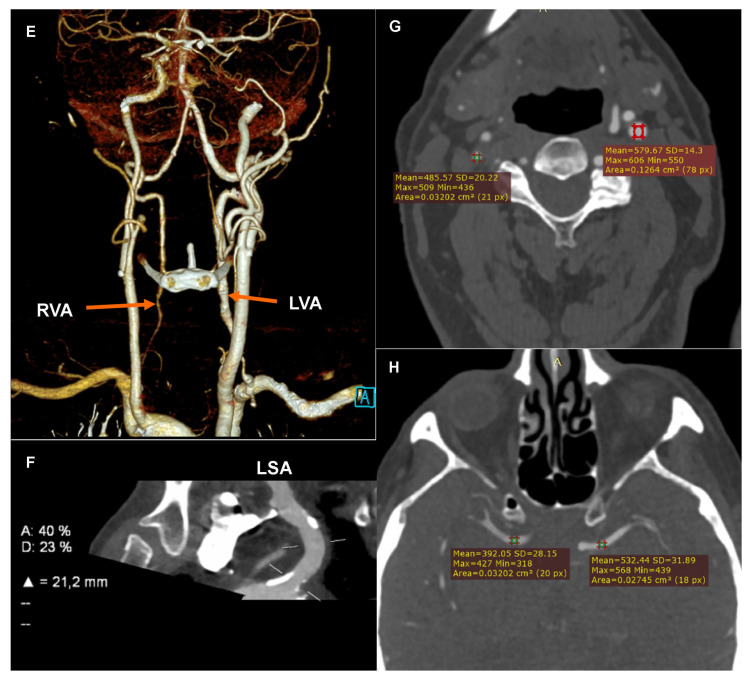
Computed tomography angiography (CTA): (A) Coronary CTA. Native phase. Axial reconstruction. Coronary artery calcium score measurement. The colors indicate the calcifications assigned to specific coronary arteries. (B) Coronary CTA. Angiographic phase. Curved multiplanar reconstruction (cMPR). Left circumflex (LCx). Measurement of the degree of stenosis. (C) Coronary CTA. Angiographic phase. Curved multiplanar reconstruction (cMPR). Left anterior descending (LAD). Measurement of the length of the myocardial muscle bridge. (D) Carotid CTA. Angiographic phase. Curved multiplanar reconstruction (cMPR). Brachiocephalic trunk (BCT). Measurement of the degree of ostial stenosis. The letter A indicates the level of vessel lumen measurement at the reference level, the letter B indicates the level of vessel lumen measurement at the level of maximum stenosis. Computed tomography angiography (CTA): (E) Carotid CTA. Angiographic phase. Volume rendering technique reconstruction (VRT). Arrows mark the recessive right vertebral artery (RVA) and the dominant left vertebral artery (LVA). (F) Carotid CTA. Angiographic phase. Curved multiplanar reconstruction (cMPR). Left subclavian artery (LSA). Measurement of the degree of stenosis. (G) Carotid CTA. Angiographic phase. Axial reconstruction. Measurement of the density of the proximal sections of the internal carotid arteries. Lower density of contrasted blood in the right carotid arteries. (H) Carotid CTA. Angiographic phase. Axial reconstruction. Measurement of the density of the cerebral arteries. Lower density of contrasted blood in the right cerebral arteries.
